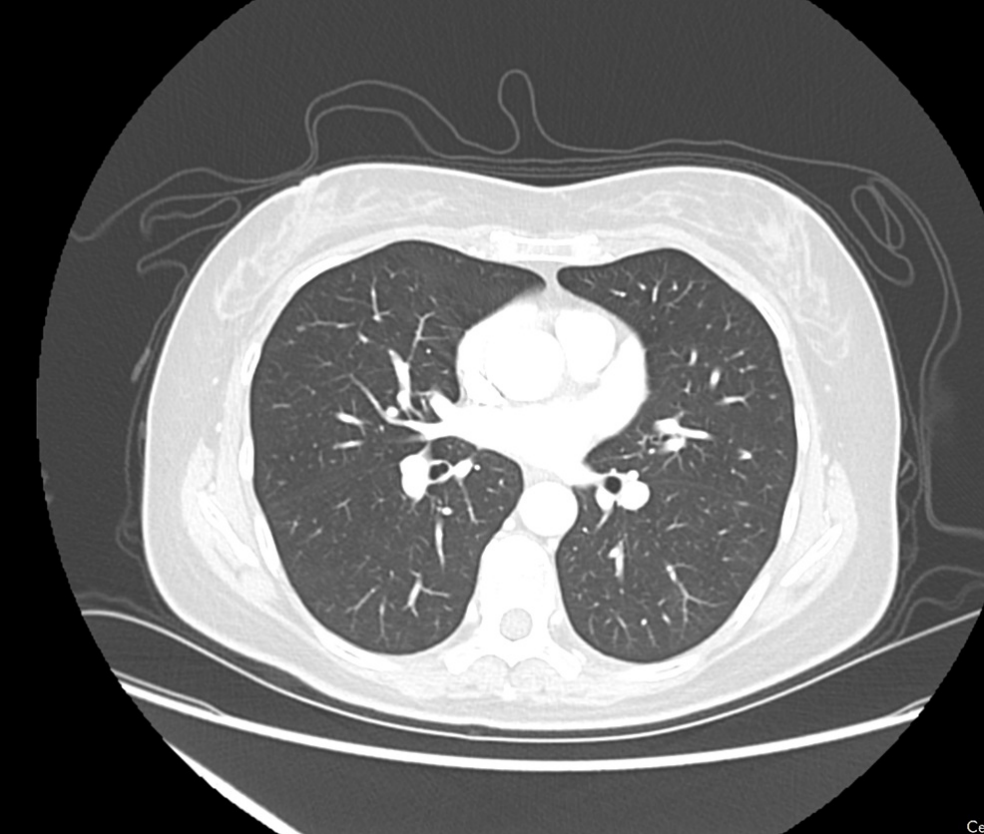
Chest CT scan shows an ill-defined asymmetrical mass in the medial aspect of the left breast.
